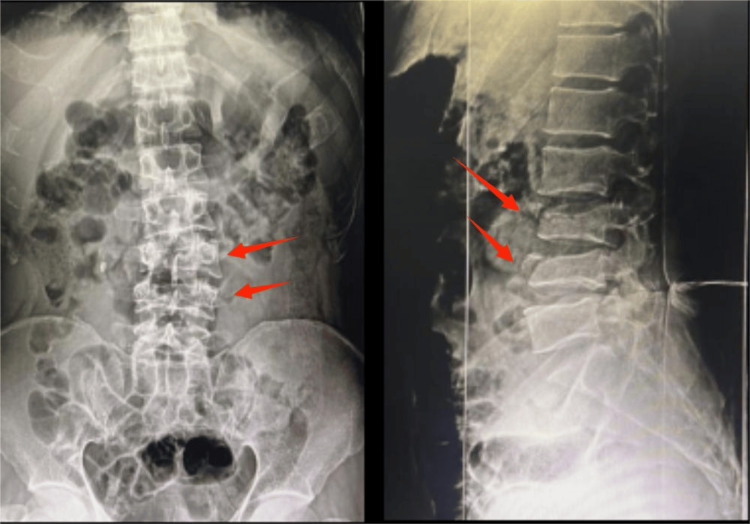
Anteroposterior and lateral views of X-ray of lumbosacral spine showing L3 and L4 burst fractures
